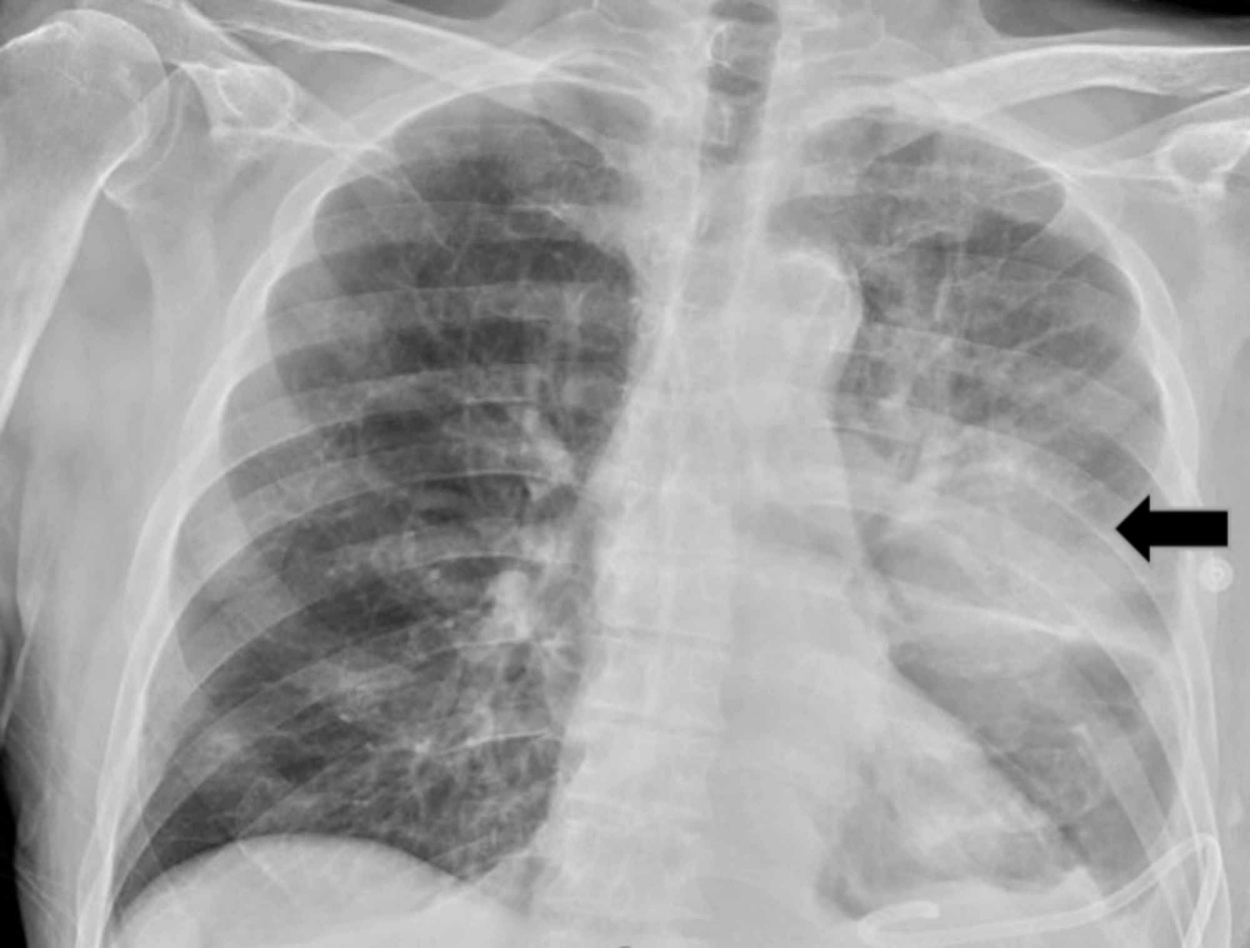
Chest radiograph demonstrating large left upper lobe lung mass (black arrow).
